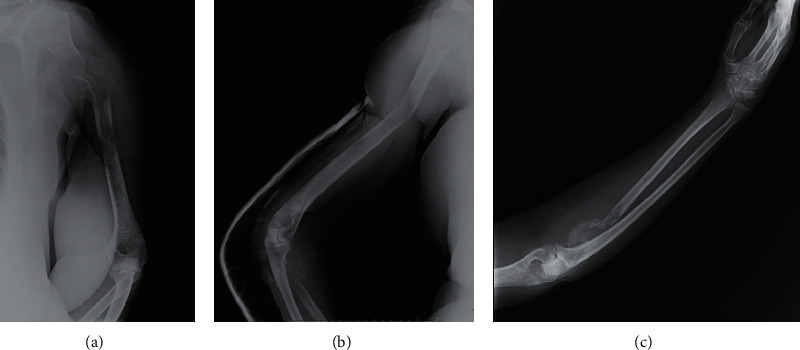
Preoperative radiographs demonstrating simultaneous lytic lesions and pathologic fractures of upper limbs secondary to a renal cell cancer.
